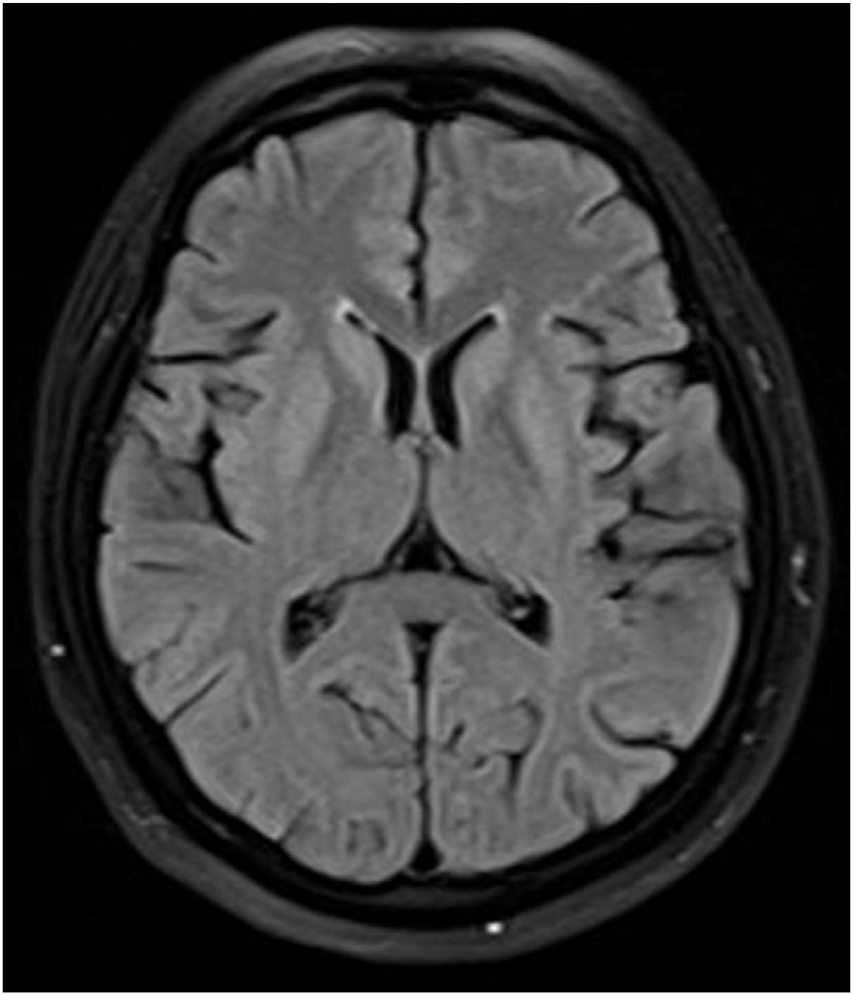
Resolution of bilateral FLAIR hyperintensities before discharge.
